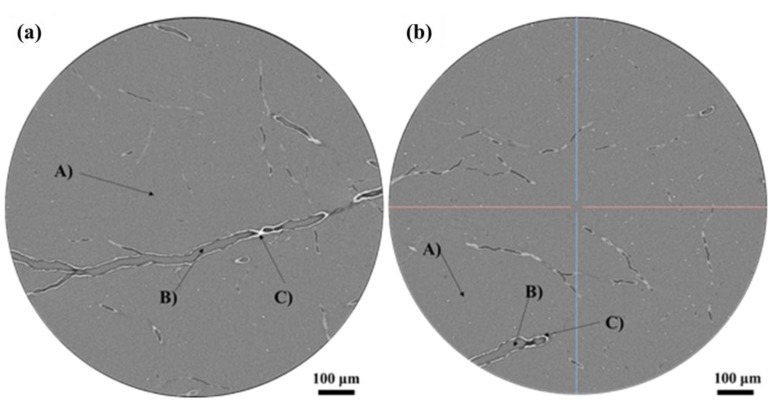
Three-dimensional-CDT (3D-CT) slice morphology (these slices are parallel to the X-Y plane in Figure 4). (a) Thicker dendritic discharge channel; (b) Thinner dendritic discharge channel.
